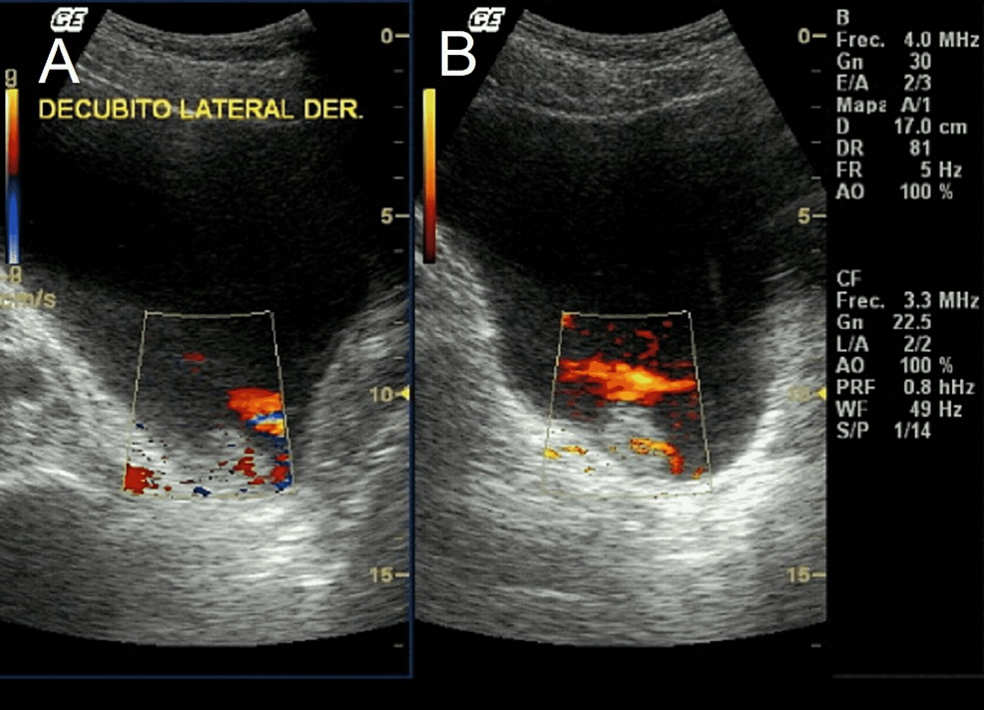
Doppler USG of the bladder showing a mass at the right bladder wall with the presence of ureteral jets on (A) sagittal view and (B) transverse view. USG: ultrasonography
